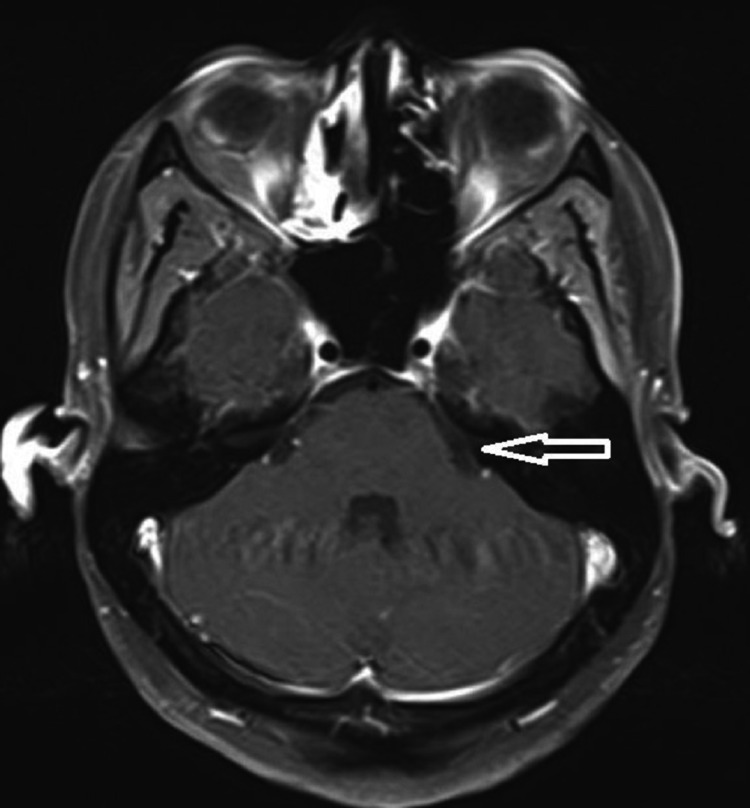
FLAIR imaging post-enhancement. The arrow points at the resolution of the prior enhancement. FLAIR: Fluid-attenuated inversion recovery
